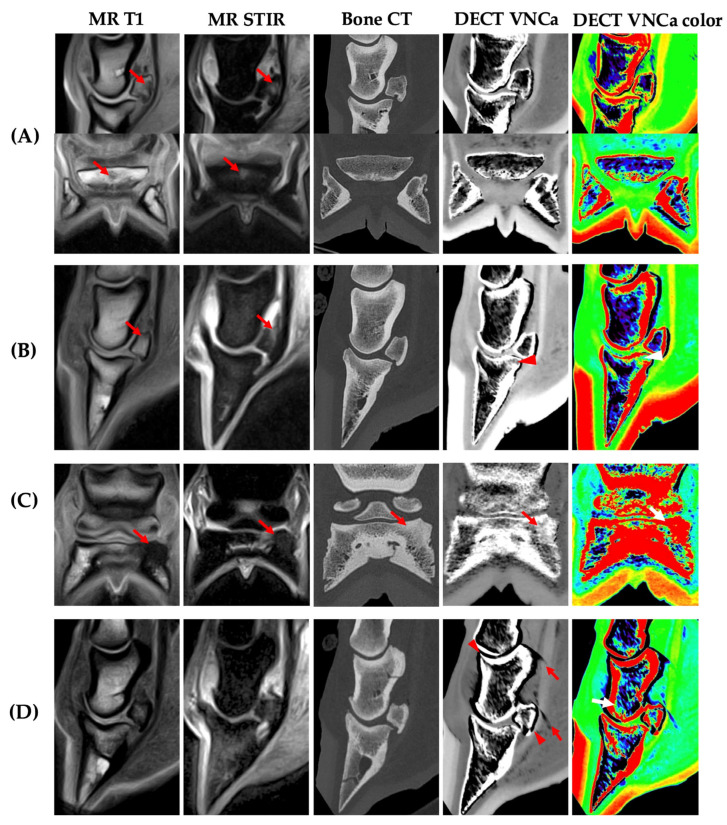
All four cases (A–D) without diagnostic agreement between DECT virtual-non-calcium (VNCa) imaging and MRI for bone edema-like lesions (BME). (A) Mild, focal, and well-delineated BME on MRI in the mid-sagittal aspect of the spongiosa and flexor cortex of the navicular bone (arrow) (sagittal and dorsal view). No sclerosis visible on standard bone CT. On the DECT VNCa map, no BME was observed. (B) Mild BME on MRI in the proximopalmar aspect of the spongiosa and flexor cortex of the navicular bone (arrow), without sclerosis on the standard bone CT. On the DECT VNCa map, readers note a false-positive BME observation at the attachment site of the impar ligament (arrowhead). (C) Moderate BME on MRI in the medial palmar process, with sclerosis of the same region on standard bone kernel CT (arrow). This sclerosis masked BME in this area on the DECT VNCa map. (D) Severe, diffuse BME in the distal phalanx and navicular bone on MRI. No sclerosis was visible on the standard bone kernel CT. On the DECT VNCa map, BME was observed; however, readers note a false-positive BME observation in the dorsodistal aspect of the middle phalanx (white arrow). Note the beam-hardening streaks (red arrow), increased noise, and volume-stitching artifact (in line with arrowheads) on the DECT VNCa map.
